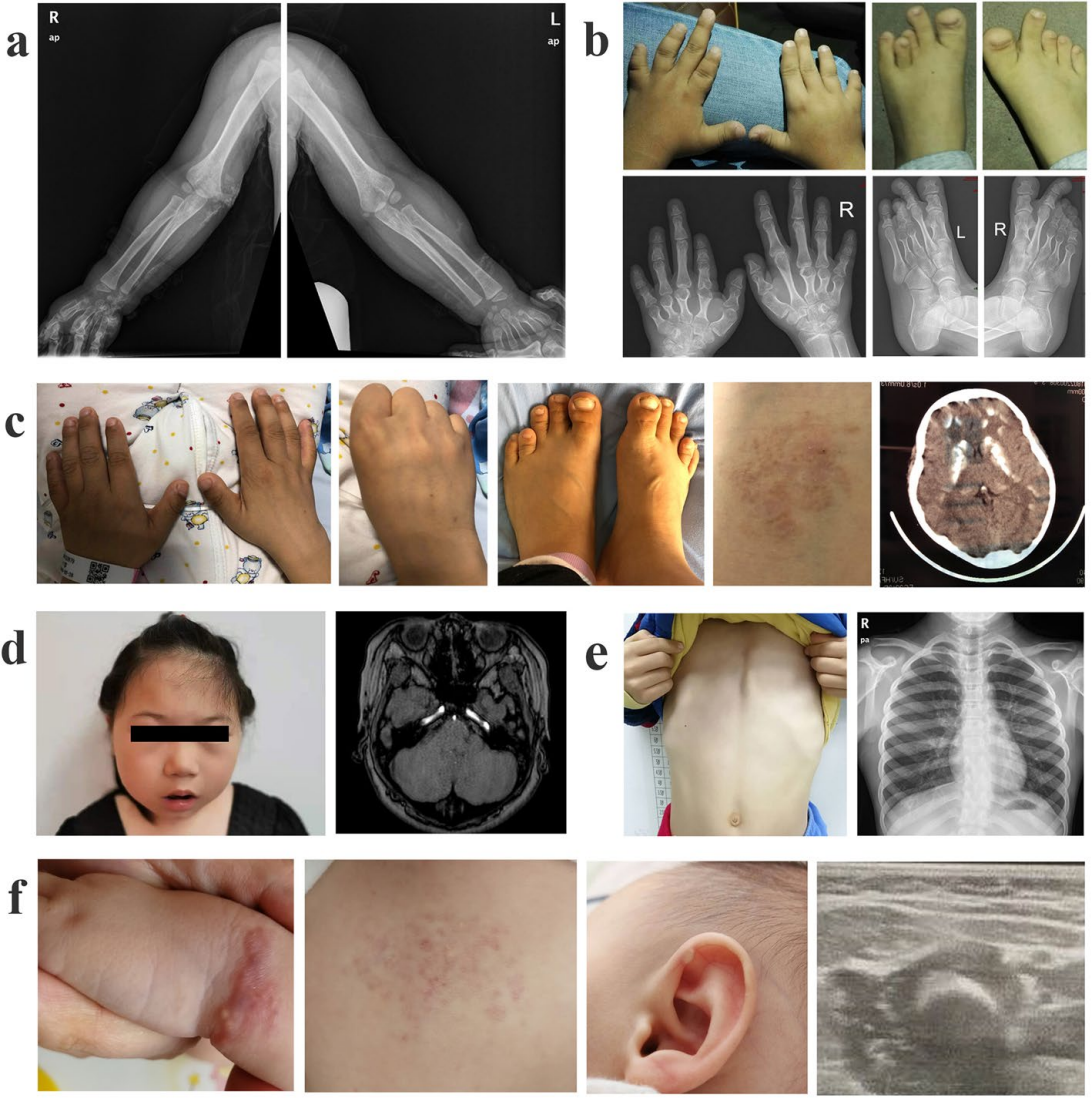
The clinical characteristics of patients with pseudohypoparathyroidism. a High density shadows in the subcutaneous soft tissue of both the wrist and right elbow were observed in patient 1.b Brachydactyly of the hand and feet digits was presented in patient 2. Shortening of the metacarpals and metatarsals was revealed by X-ray, involving F2-5 on the left, F3-5 on right, and T3-4 on both sides. c Brachydactyly of the hands and feet was noted in patient 3. Radiographs of patient 3 revealed shortening of the metacarpals and metatarsals, particularly F1-5 and T4 on both sides. Patient 3 also presented with subcutaneous calcifications across their back, and cranial CT revealed multiple bilateral calcifications involving the cerebral hemisphere. d Patient 6 presented with a rounded face, and her head MRI revealed basal ganglia calcification. e Patient 9 experienced deformity of thorax, and her chest radiograph revealed scoliosis. f Patient 10 suffered from subcutaneous calcifications in the left thigh with developing nodes in the left wrist, both shanks, the back, the abdomen, the auricula, and the inframandibular region. Radiograph revealed high density shadows in both lower limbs
